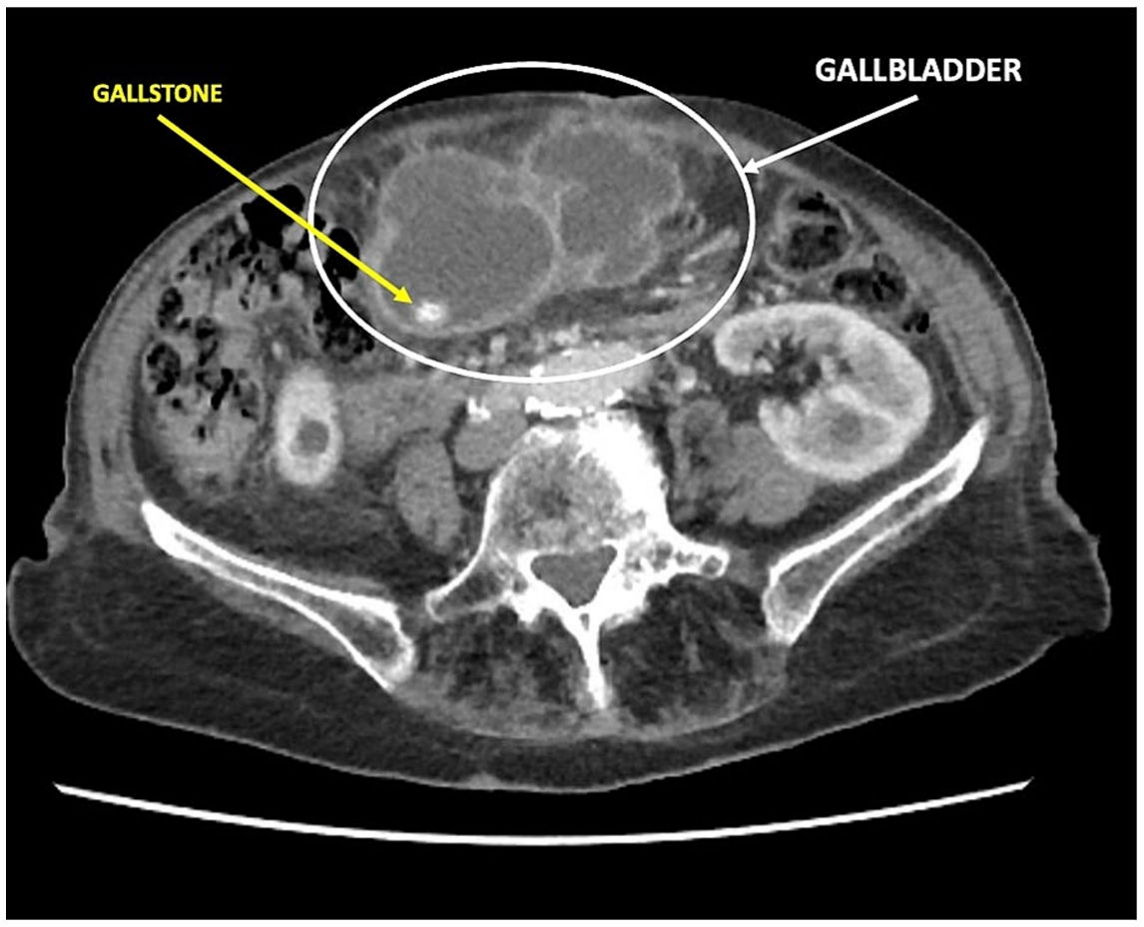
CT scan during first admission showed a markedly distended gallbladder extending into the mesogastric region, without hernial orifice involvement.
